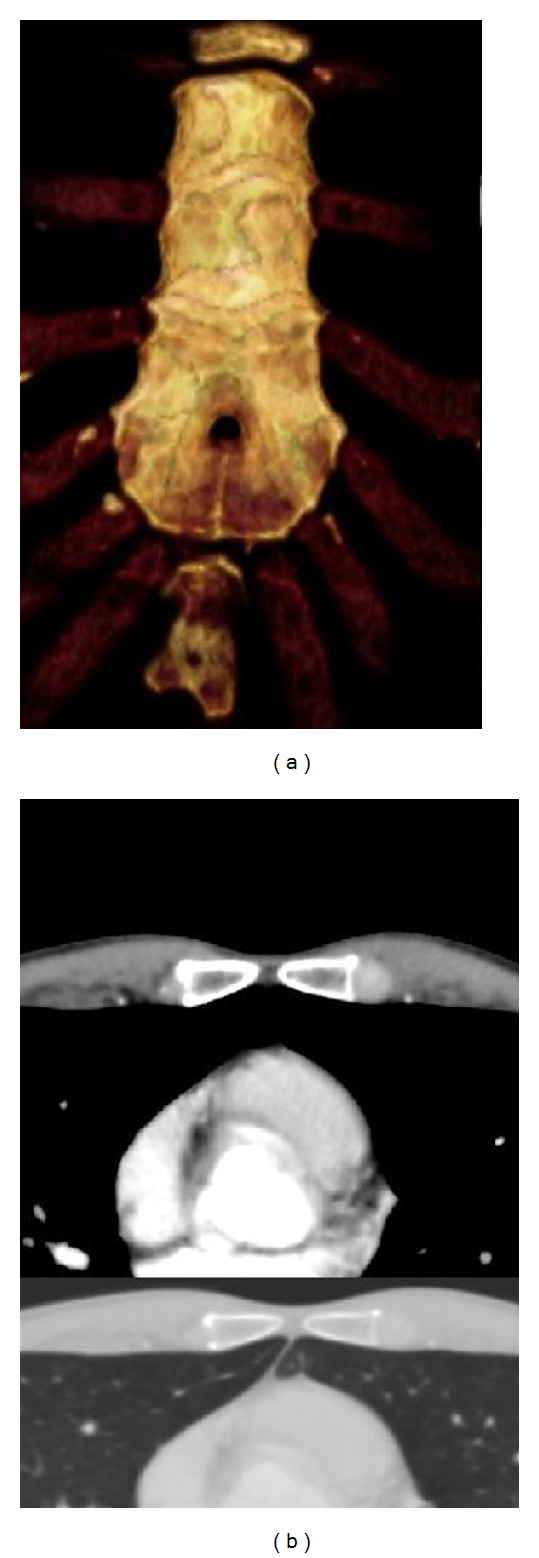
Computed tomographic images of a typical sternal foramen. The axial slices (b) are showing the close contact to vital structures (lung and heart). There is a potential risk of life-threatening complications (pneumothorax/cardiac tamponade), if a needle is inserted unintentionally through this foramen.
